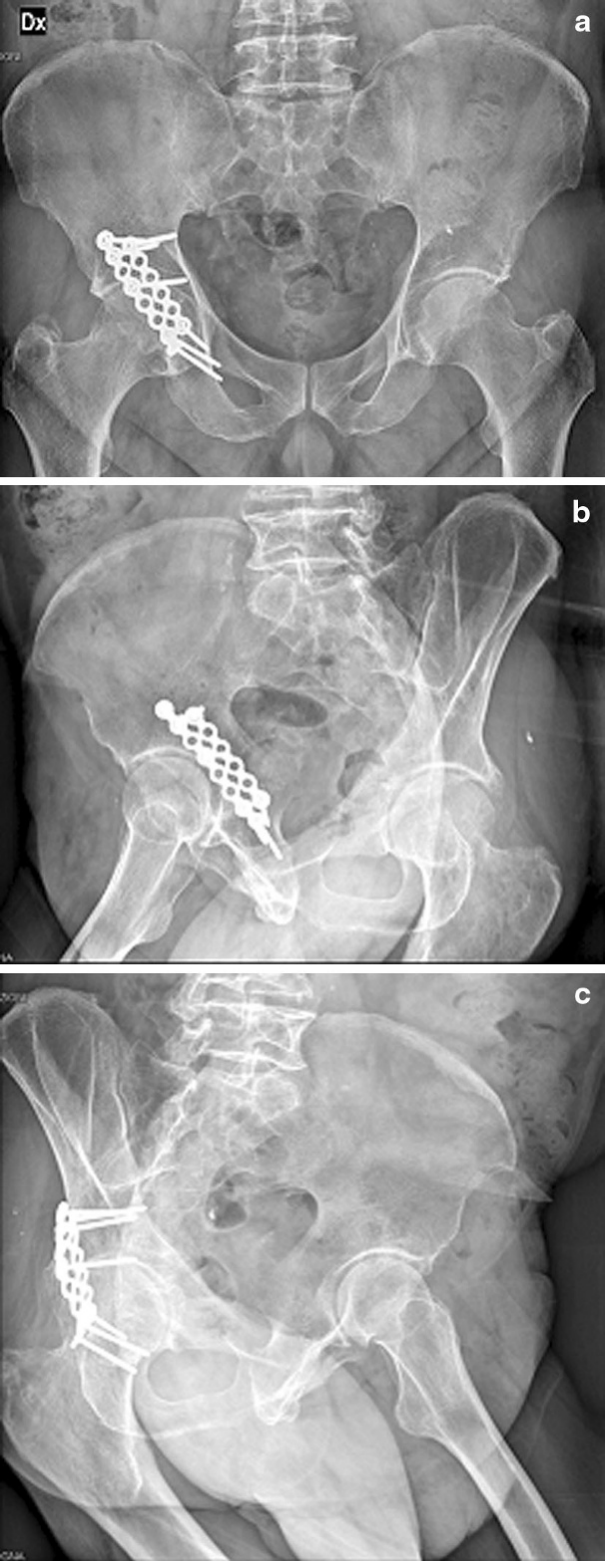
X-rays at the last follow-up of the same patient as in Fig. 1 (May, 2013): a AP and b, c oblique Judet views
